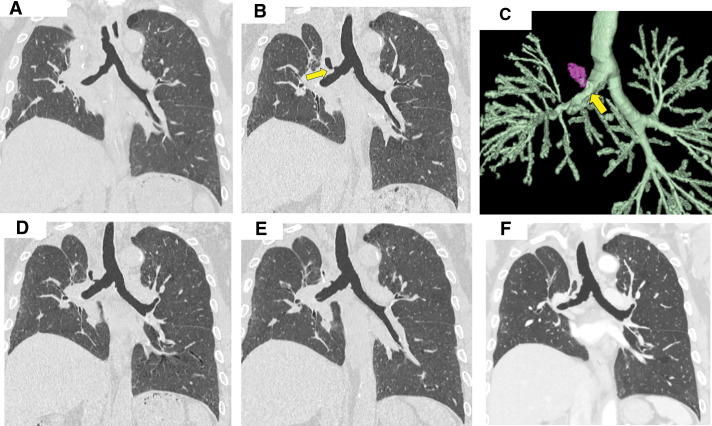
Postoperative CT scans obtained before and after the diagnosis of BPF. (A) CT scan on POD 18 showed pneumonia in the right S6 segment, without any abnormal findings at the bronchial stump. (B) CT scan on POD 32. The right upper bronchial stump communicated with a cystic structure at the right bronchial hilum (yellow arrow). (C) 3D CT on POD 32 demonstrating direct communication between the bronchial stump (yellow arrow) and the hilar cystic cavity (purple area). (D) CT scan on POD 55 showed a reduction in the size of the cystic lesion. (E) On POD 69, the BPF was almost closed. (F) CT scan on POD 206 demonstrated complete closure of the BPF without evidence of bronchial stenosis or residual air space. BPF, bronchopleural fistula
